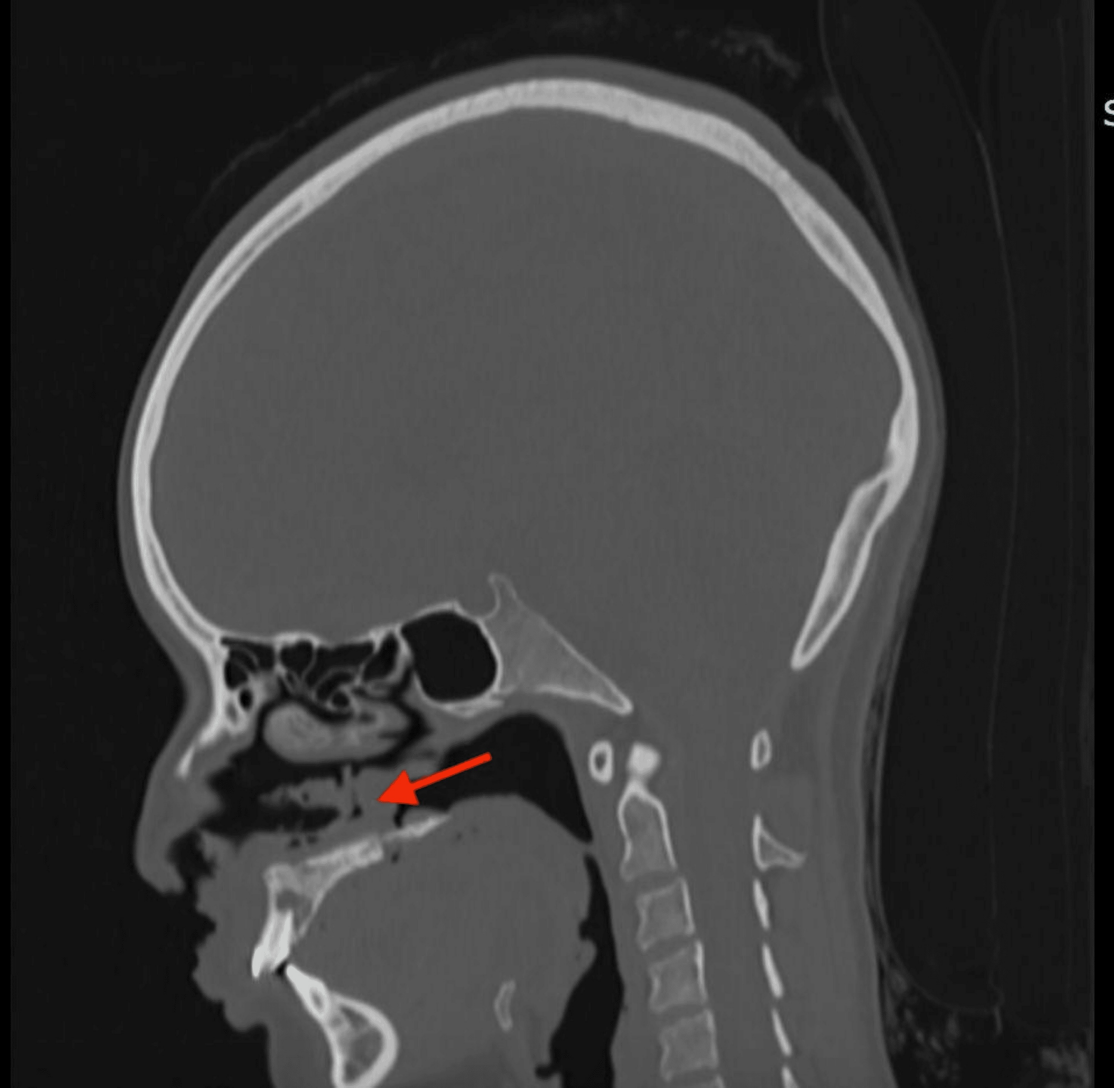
CT scan Sagittal computed tomography: Disruption of soft tissues in the left upper labial region, nasolabial fold, and nasal ala, predominantly on the left side, with perforation of the cartilaginous portion of the nasal septum. There was also the presence of material with soft-tissue density and a gaseous appearance in the nasal and oral vestibule, secondary to perforation of the soft palate (red arrow). Bone tissue shows areas of sclerosis and lytic lesions in the alveolar and palatine processes of the maxillary bone, affecting the posterior surface of the upper incisors, the anterior surface of both maxillary bones, and the horizontal plate of the palatine bone.
